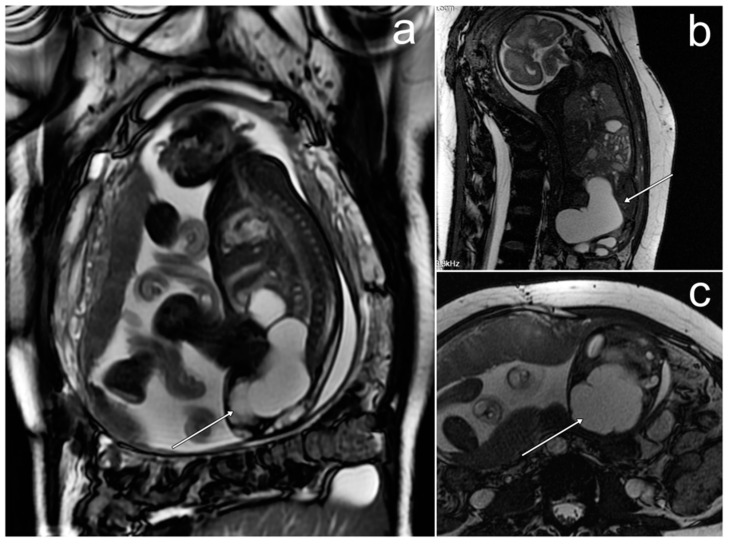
A fetal MRI. A singleton pregnancy, 29th gestational week (GW). A predominantly cystic sacrococcygeal teratoma, mainly located outside the body, a minor part of it in the pelvis, adjacent to the sacrum—type III, according to the classification of the Section of Pediatric Surgery of the American Academy (a–c, arrows). (a–c)—2D/FIESTA.
